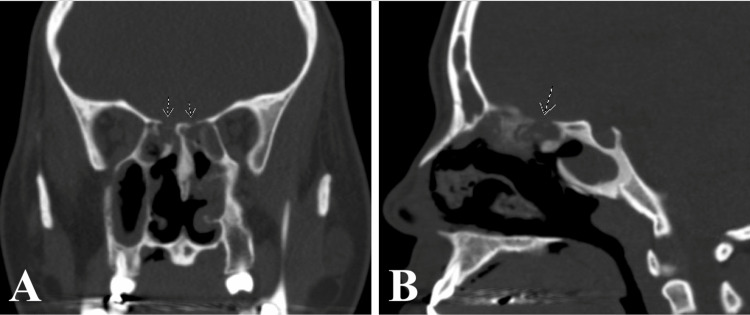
CT of the sinuses in AP (A) and lateral (B) views with evidence of diffuse tissue destruction Arrows point to destructive bone lesions CT: computed tomography; AP: anteroposterior
